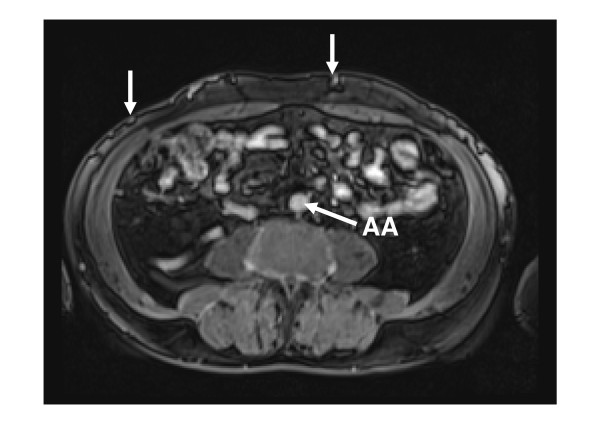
Gadolinium-enhanced magnetic resonance imaging (MRI) of the abdomen at infrarenal level showing abdominal wall veins and absence of the inferior vena cava. AA, abdominal aorta
